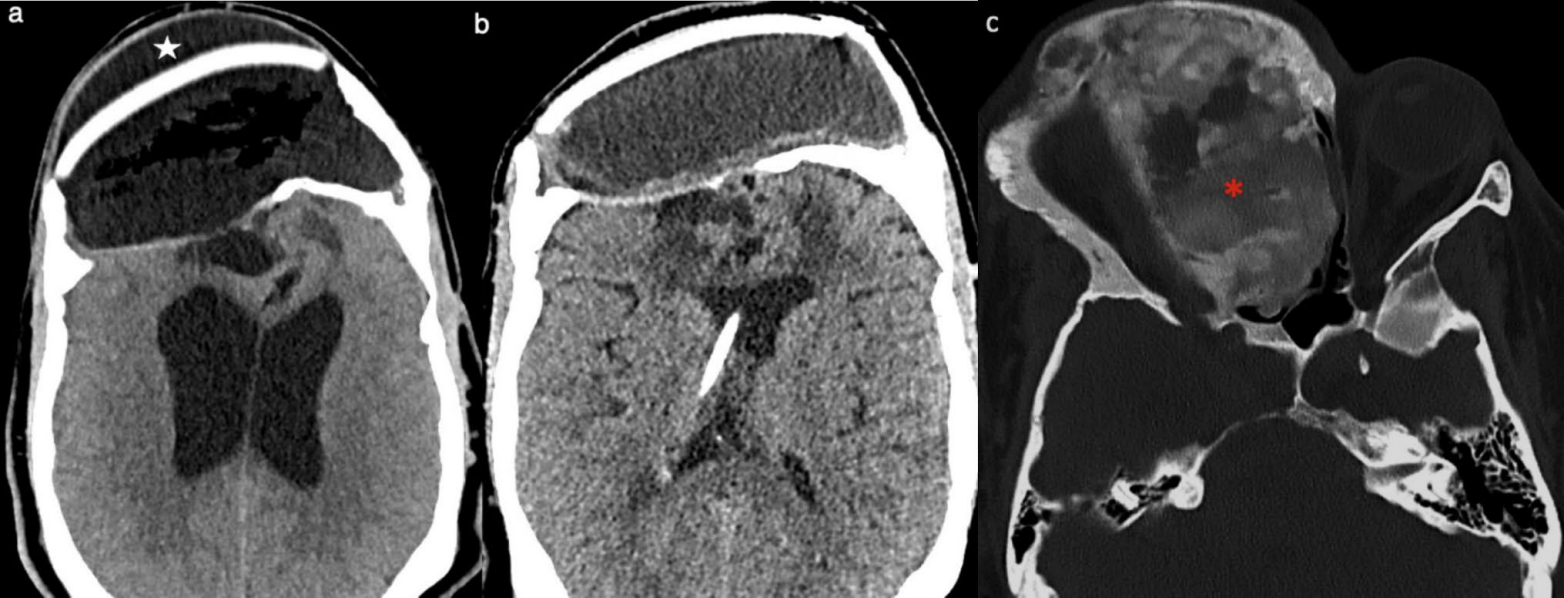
Follow-up images. a Axial CT scan 5-months after surgery demonstrated dilatation of the lateral ventricles and pseudomeningocele. The white star highlights the cerebrospinal fluid collection around the cranioplasty. The patient underwent a ventriculoperitoneal shunt placement. b Axial CT scan after 4-years showed significant decrease in ventricular size, and stable postoperative changes. c Axial CT scan with bone window settings revealed that the untreated disease remained stable
